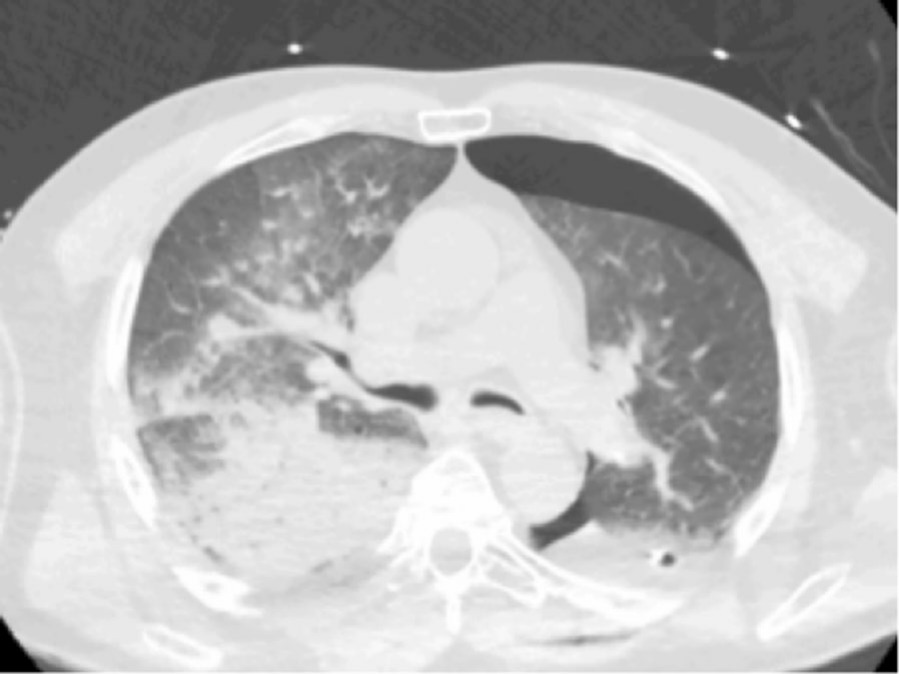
Non-cardiogenic pulmonary oedema. Axial CT image (parenchymal window) showing an ARDS after left lower lobe segmentectomy. In this case, ARDS abnormalities were predominant on the right side; gravito-dependent pulmonary condensations are present with normal anterior lung, then ground-glass opacities, then posterior alveolar condensationsI.
